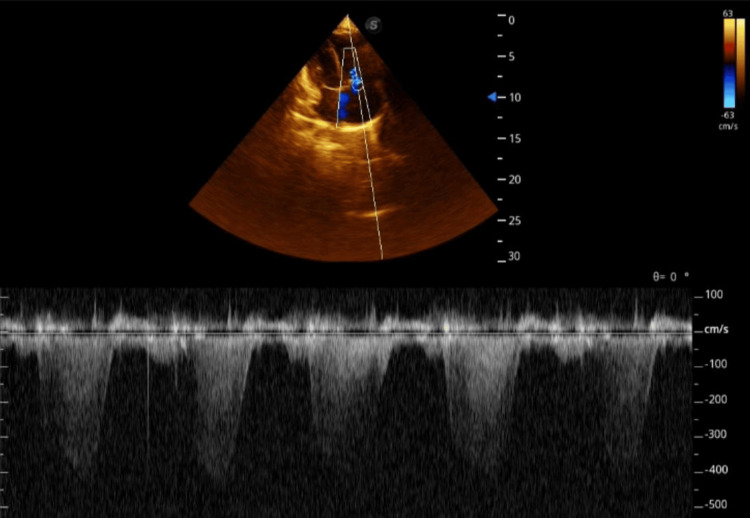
Continuous wave Doppler on the tricuspid valve
